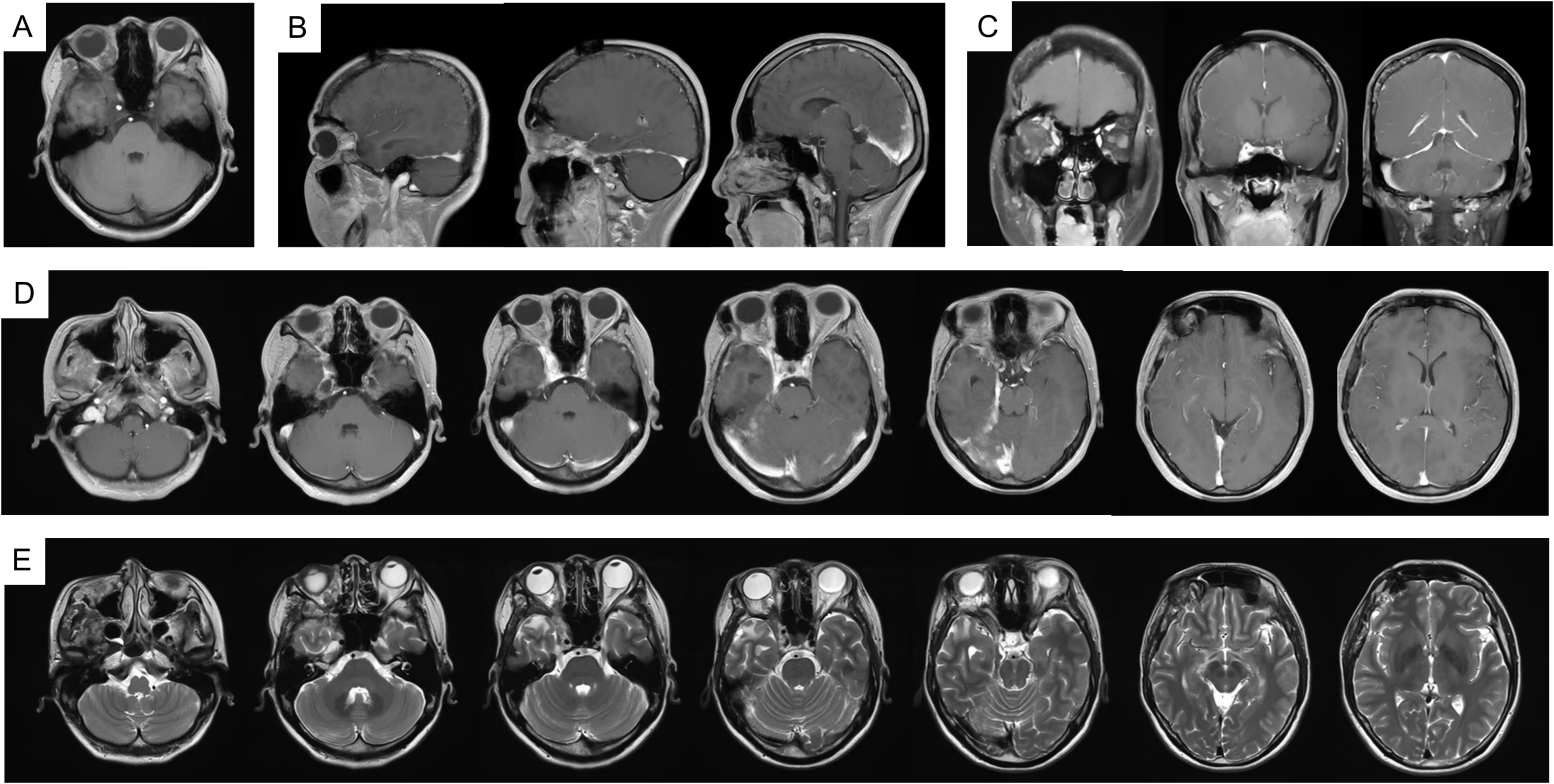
MRI imaging at 13 months follow up radiology. (A) T1WI; (B) T1WI-C coronal view; (C) T1WI-C sagittal view; (D) T1WI-C; (E) T2WI-TSE.
